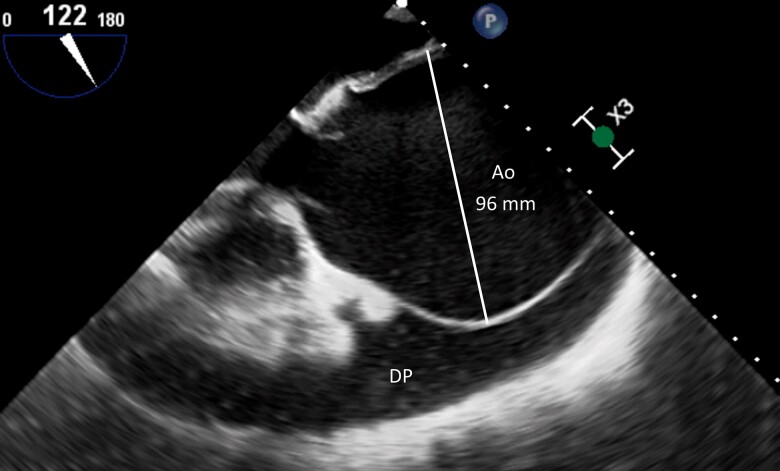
Post-pericardiocentesis transoesophageal echocardiogram in the mid-oesophageal view showing a large ascending aortic aneurysm (Ao) and residual pericardial effusion (PE).
